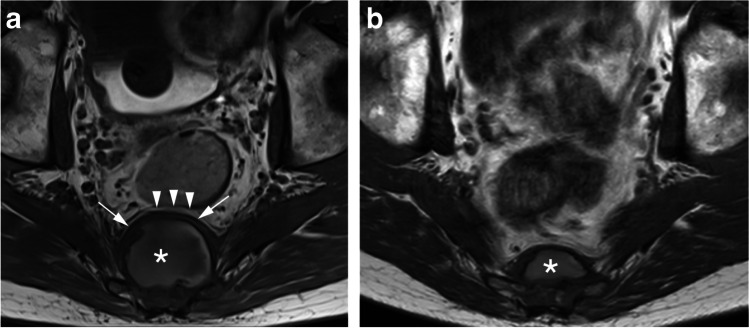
Baseline (a) and follow-up (b) axial non-contrast T1-weighted images. a Presacral soft tissue component with a native hyperintense signal centrally (*) and a thick hypointense rim on the anterior side (white arrows). The lesion causes a mild mass effect over the rectum with a preserved fat plane in between the tumor and the rectum (arrowheads). b Note the marked decrease in size occurring in 4 weeks time. The signal intensity in the soft tissue mass has not changed (*)
